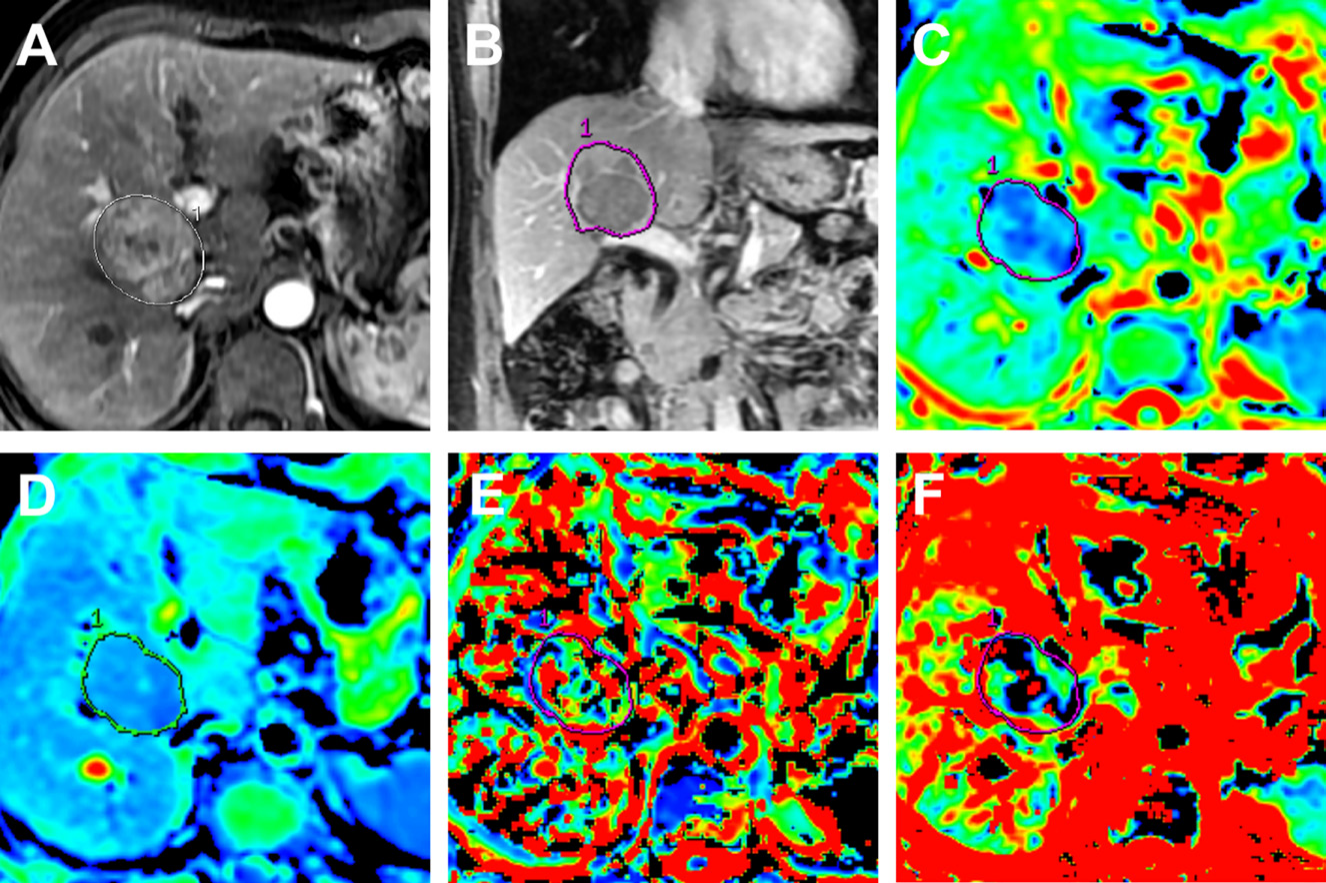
MR images in a 58-year old man with surgically confirmed HCC (A) Arterial phase MR image. (B) Portal venous phase MR image. (C) Standard ADC map. (D) ADC_slow_ map. (E) ADC_fast_ map. (F) *f* map. The tumor shows typically hyperintense on arterial phase MR image and hypointense to liver parenchyma on portal venous phase. The ADC map demonstrates a lower ADC value (0.98 × 10^−3^ mm^2^/s) compared with cutoff value (1.18 × 10^−3^ mm^2^/s), the ADC_slow_ also demonstrates a lower ADC value (0.76 × 10^−3^ mm^2^/s) compared with cutoff value (1.06 × 10^−3^ mm^2^/s).
